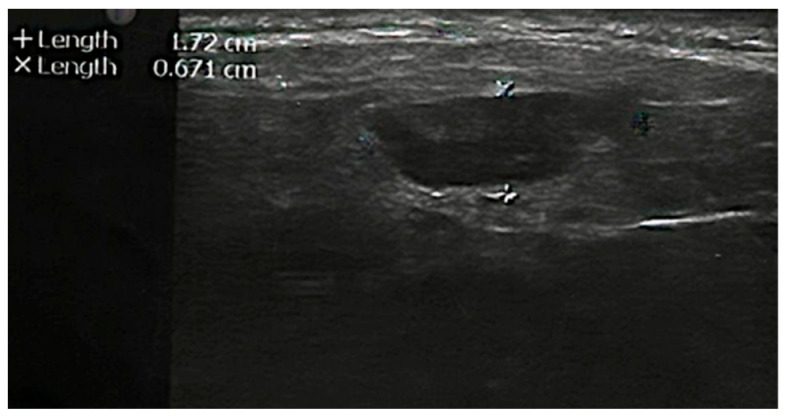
Ultrasonography shows the well-circumscribed tumor in the left parotid.
